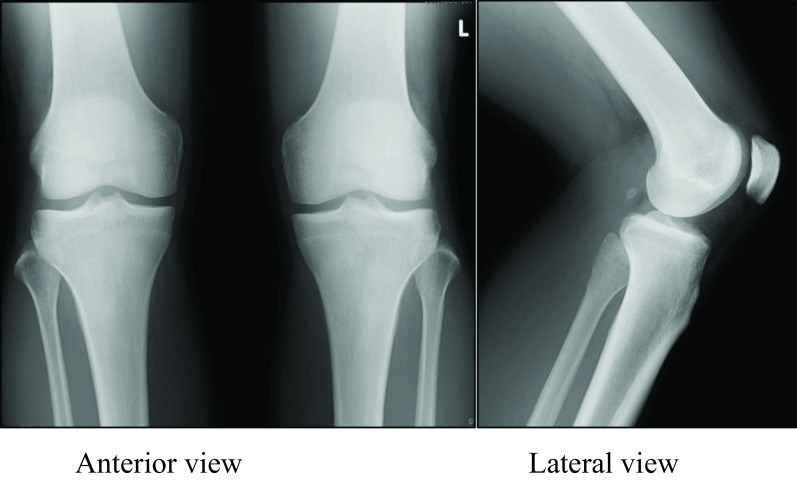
Plain X-ray images at the first consultation. Inclination angle of proximal tibiofibular joint was 26° (based on the measurement method of Ogden), showing an oblique type, with no notable articular degeneration of the proximal tibiofibular joint.
